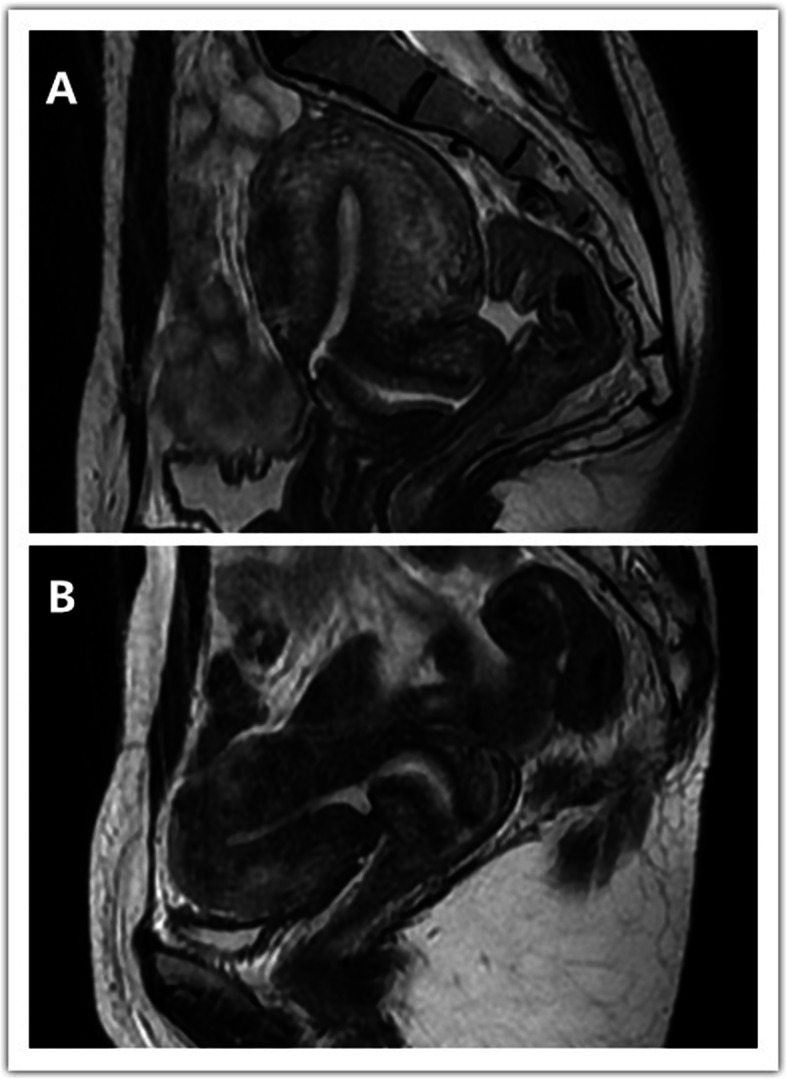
MRI scans of cesarean scar defects with adenomyosis. A. Sagittal view on T2 images (retroflexed uterus). B Sagittal view on T2 images (anteflexed uterus)
